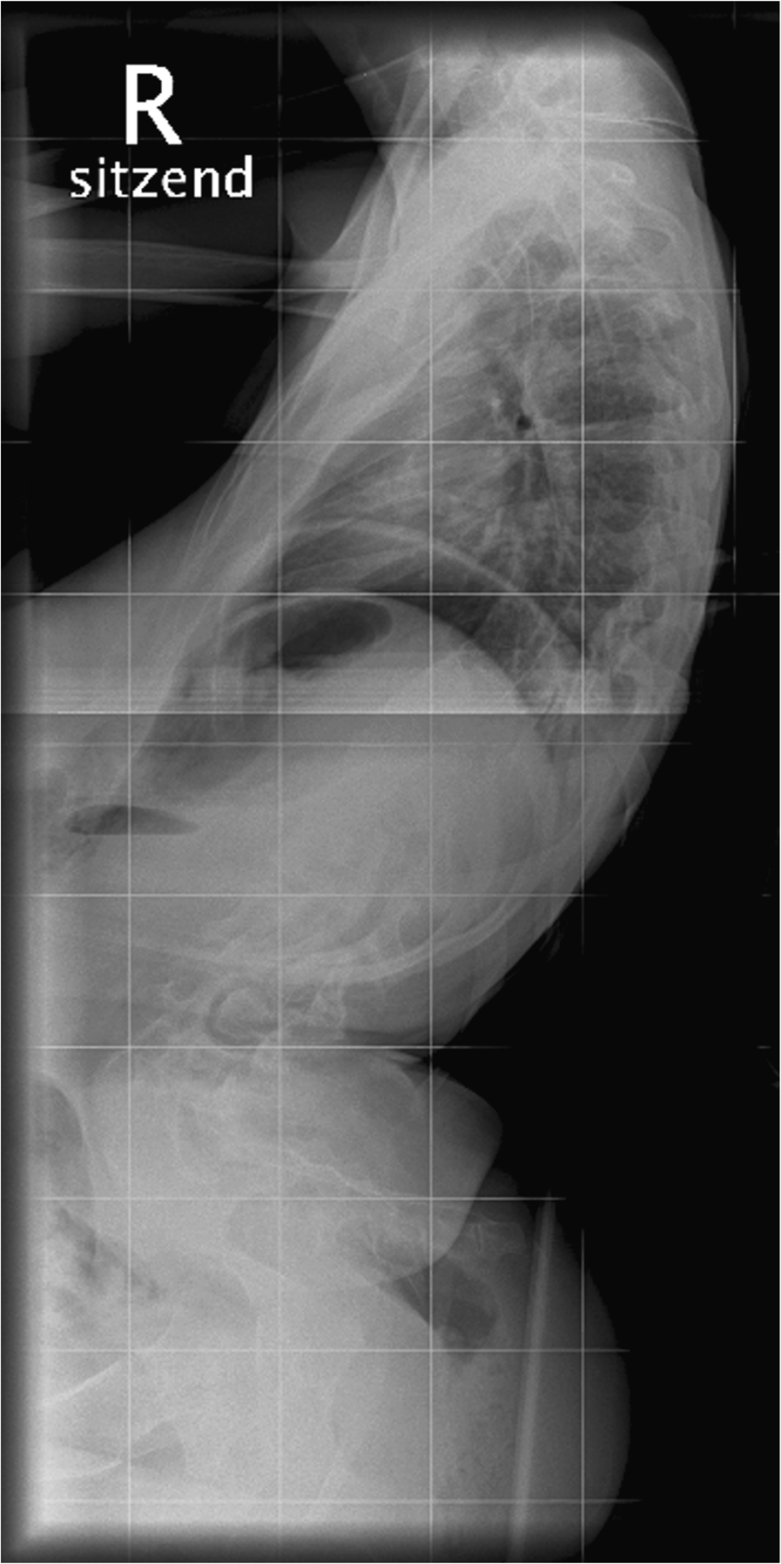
Lateral preoperative X-ray of the spine: Cobb angle of 100° (Th8-L1-L5) left-convex , 45° pelvic tilt to the left.
